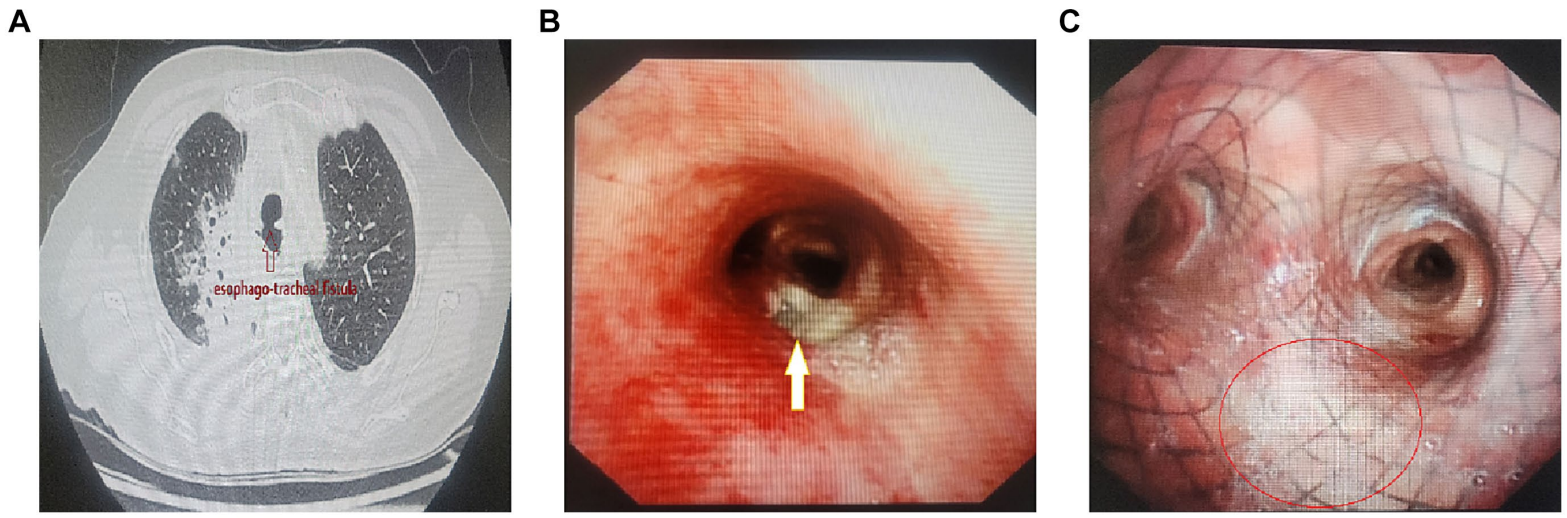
(A) CT shows esophago-tracheal fistula (↑) with aspiration pneumonia of the right lung. (B) Bronchoscopy reveals a 2 cm diameter esophageal-tracheal fistula adjacent to the right main bronchus in close proximity to the carina (↑). (C) The fistula (○) was sealed by self-expanding Y-shaped metal covered stent.
